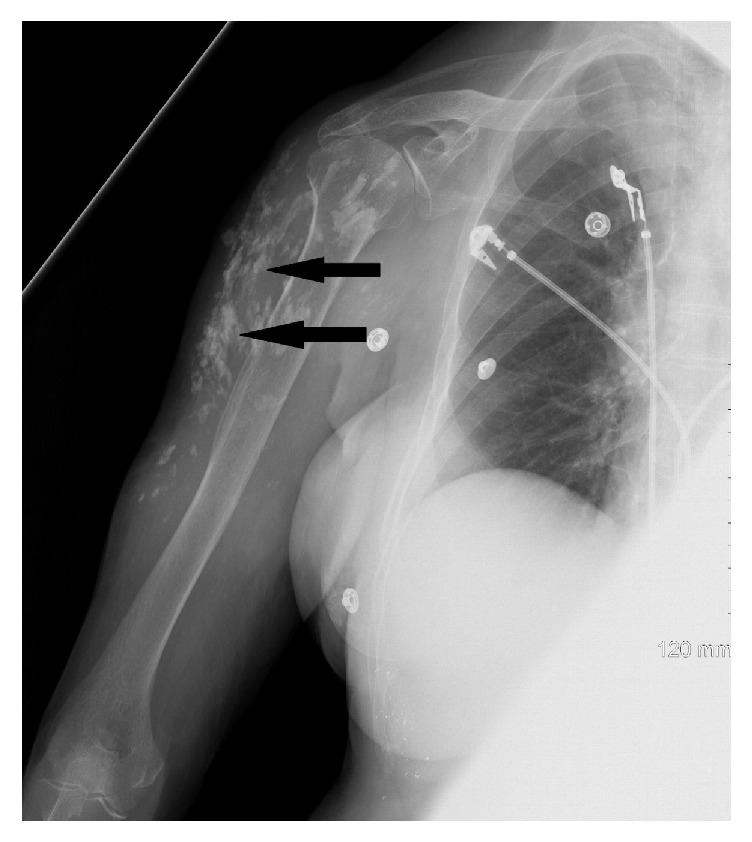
X-ray of the right arm showing soft tissue calcifications.
